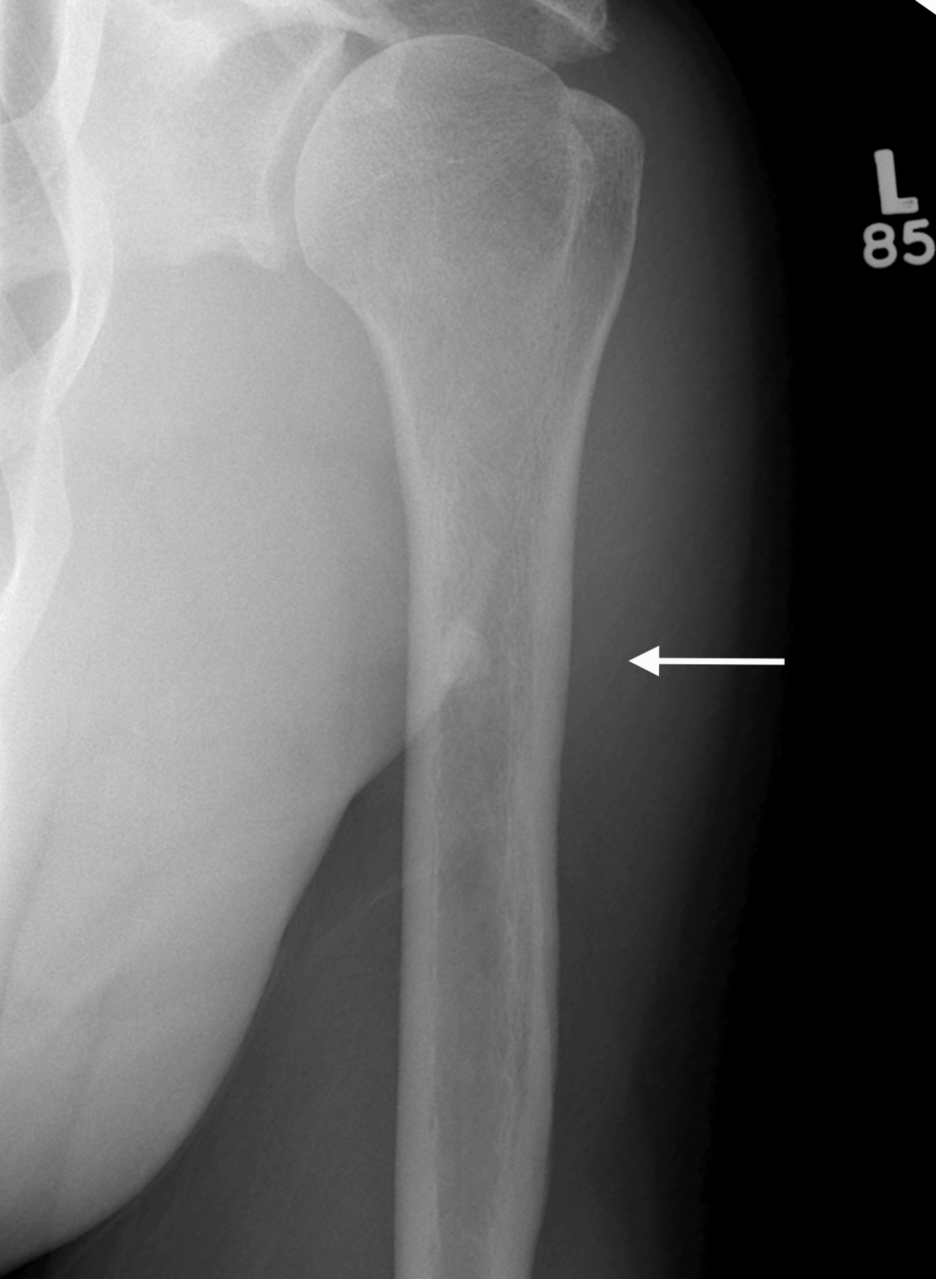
Left shoulder X-ray showing a small medullary infarct (white arrow) at the medial aspect of the proximal humeral shaft
